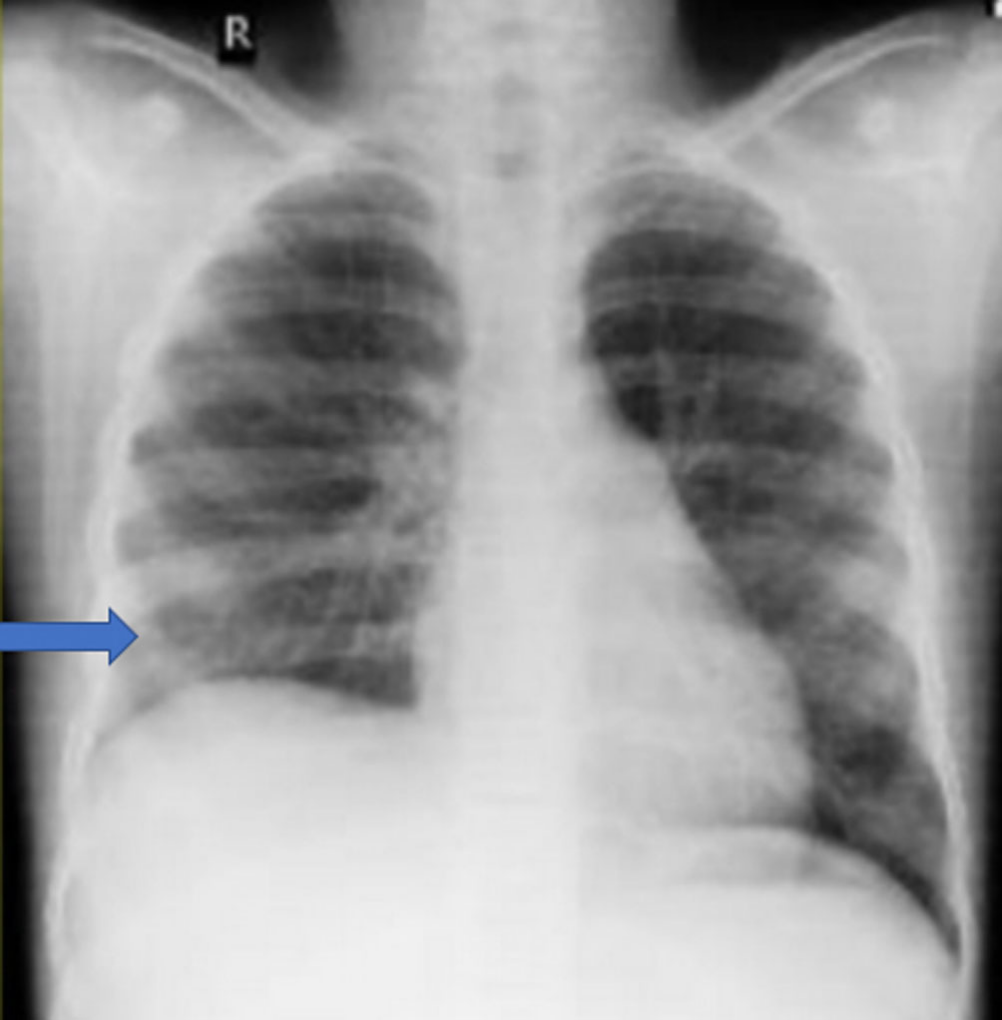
Chest X-ray showing complete resolution of pleural effusion after four weeks.
